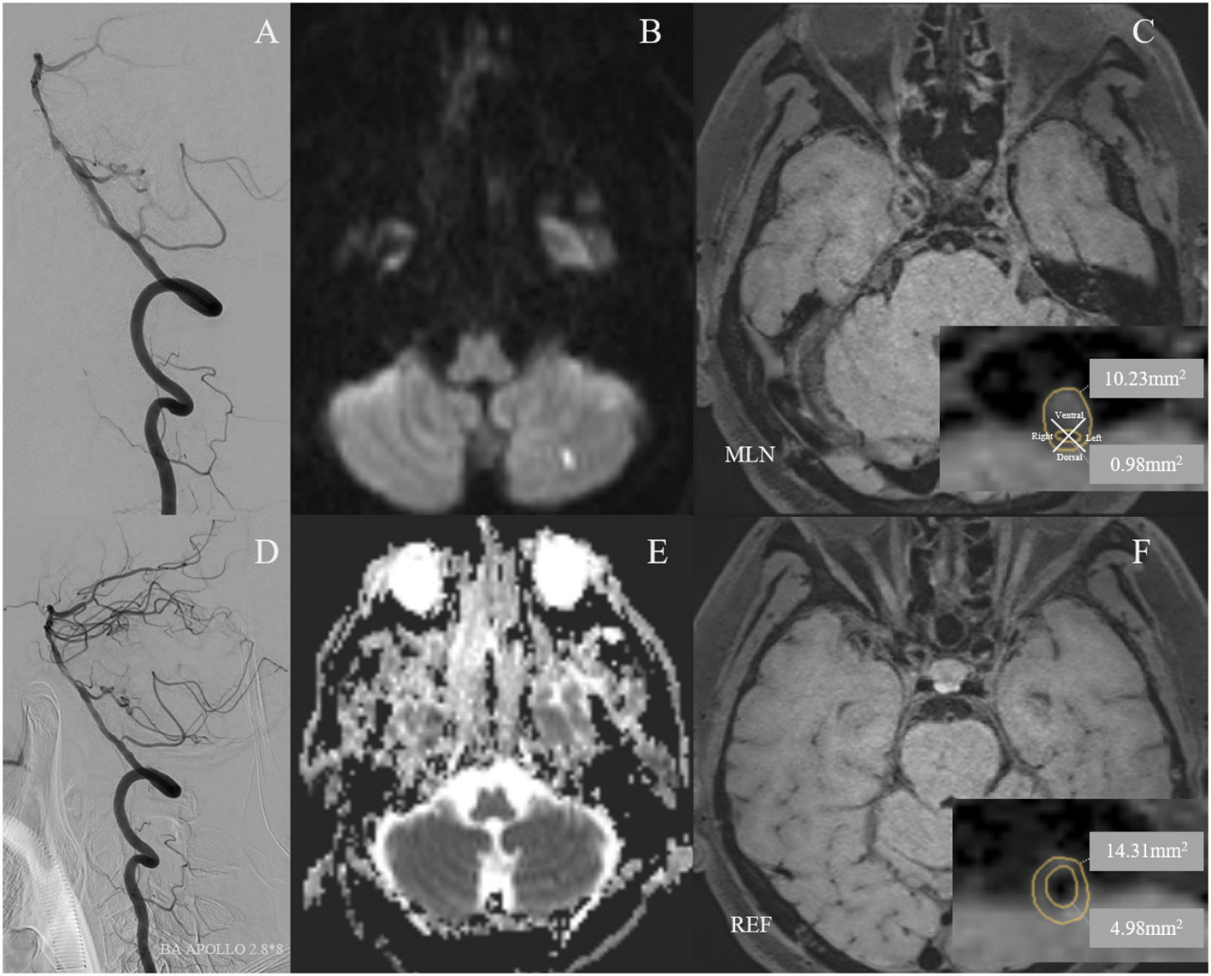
A 59-year-old man with cigarette smoking history and drinking history presented with recurrent transient attacks of dizziness for 2 months. (A) Pre-operative digital subtraction angiography (DSA) showed stenosis at the middle segment of the basilar artery (BA) with 75.5% of degree. (D) A 2.8 × 8 mm Apollo balloon-mounted stent (MicroPort NeuroTech, Shanghai, China) was placed at the stenotic segment. The patient had a new cerebral infarction at the left cerebellum within 72 h after stenting without clinical symptoms, which was detected by diffusion-weighted imaging with a new high signal (B) and apparent diffusion coefficient imaging with a new low signal (E). Figures of the right column are cross-sectional T1-weighted BA images at the maximal lumen narrowing (MLN) (C) and reference (REF) (F) sites. The plaque was eccentric and belonged to the ventral side of the BA (C). Vessel area (VA) and lumen area (LA) at the MLN (C, VA 10.23 mm^2^, LA 0.98 mm^2^) or REF (F, VA 14.31 mm^2^, LA 4.98 mm^2^) sites were manually traced for measuring after zooming in 400 times. Wall area (WA) at the MLN or REF sites was calculated by VA-LA. The plaque burden was calculated as [(WA _MLN_-WA _REF_)/VA _MLN_] × 100% and the remodeling index was calculated as VA _MLN_/VA _REF_. Therefore, the plaque burden was −0.78%. The remodeling index of the vessel at MLN was 0.71, which was categorized as negative remodeling.
